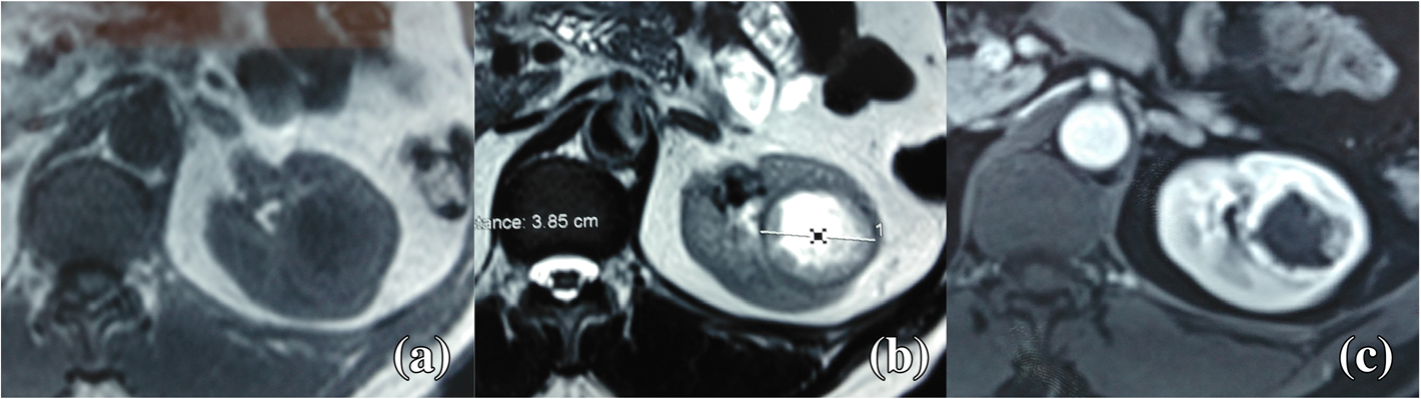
Magnetic resonance imaging axial view shows a hypointense mass on T1 (a) and hyperintense on T2 (b) with intense peripheral enhancement (c)
